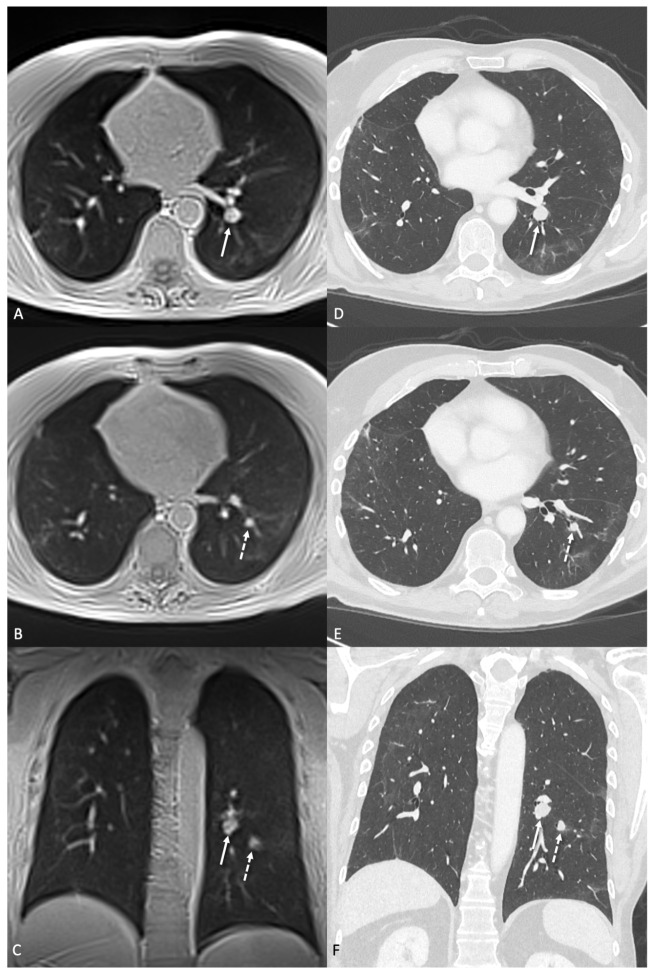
Two metastases of a malignant melanoma in the left lower lobe. The bigger nodule (arrow) with 16.7 × 13.6 mm is next to the left hilus vessels but clearly visible in transversal (A) and coronal (C) ultra-short echo time (UTE) sequences. The second is located a bit more caudally and has a size of 11.2 × 7.4 mm (dashed arrow) but is also clearly visible on MRI (B,C). (D–F) show the corresponding CT images with 1 mm slice thickness.
